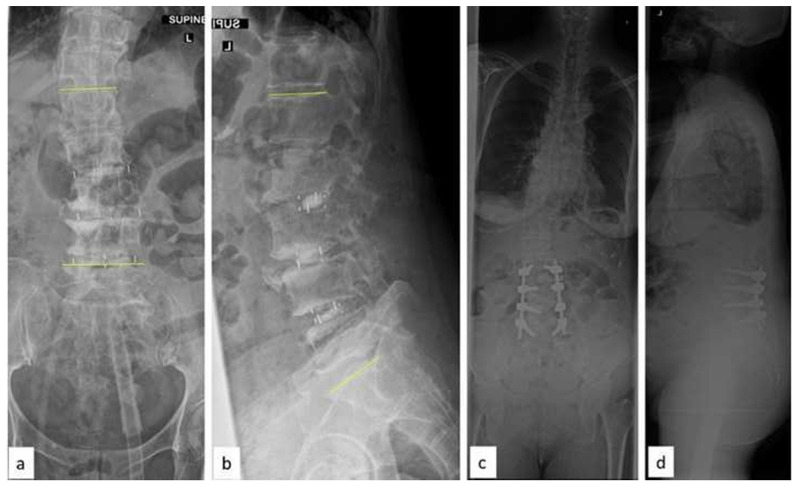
(a–b) Supine AP and Lateral radiographs after first-stage surgery. Note the correction of coronal Cobb’s and optimal lordosis using L2–L5 OLIF cages (c–d) Post-operative whole spine AP and Lateral radiographs after second stage surgery (L2–L5 MIS posterior instrumentation).
